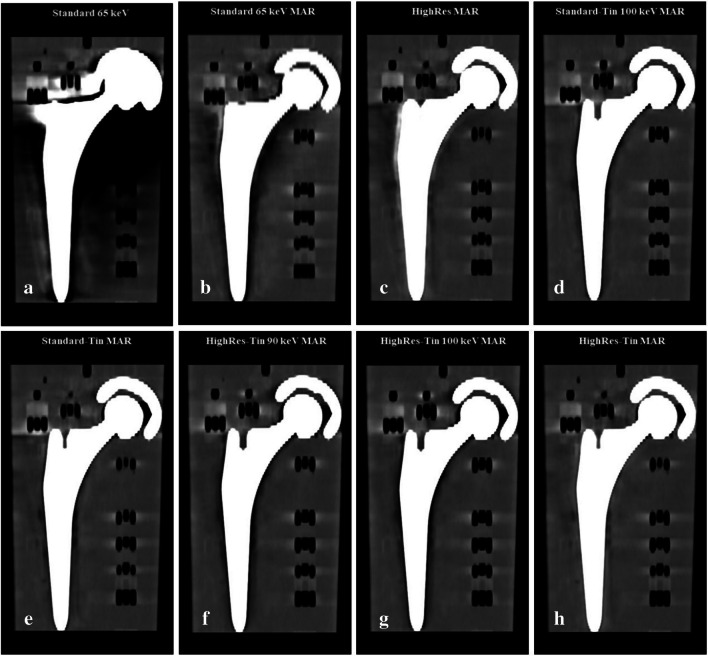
Coronal PCD CT images acquired with (a) Standard 65 keV (factory preset); b Standard 65 keV MAR; c HighRes MAR; d Standard-Tin 100 keV MAR, (e) Standard-Tin MAR; f HighRes-Tin 90 keV MAR; g HighRes-Tin 100 keV MAR; and (h) HighRes-Tin MAR. The selected images (b-h) with the parameters described above consistently achieved superior image quality compared with the reference image (a) and were rated equally as the best in terms of overall image quality. Standard = Quantumplus; Standard-Tin = QuantumSn; HighRes = UHR Q + ; HighRes-Tin = UHR QuantumSn; PCD CT = photon-counting detector computed tomography; Sn = tin filter; UHR = ultra-high resolution; VMI = virtual monoenergetic imaging
